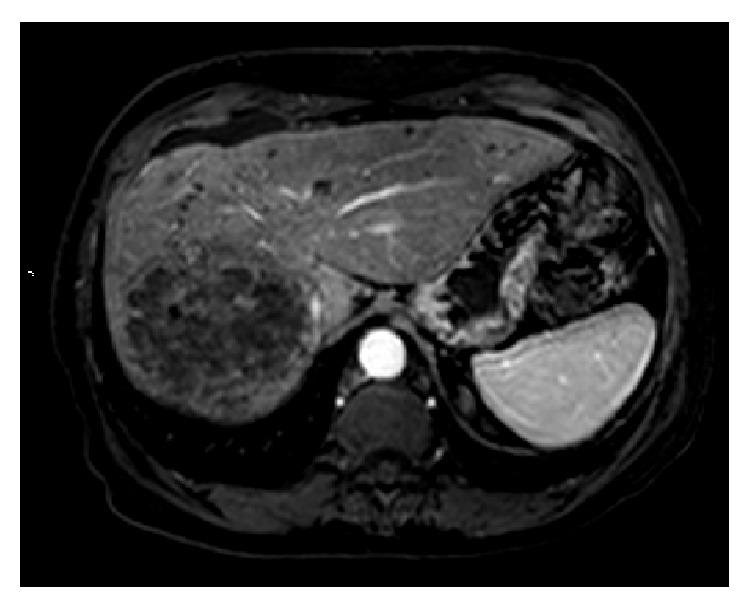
Same lesion on MRI 2 months following radioembolization with yttrium 90 resin microspheres (09/20/2013). There is no enhancement within the central portion of the mass and the tumor has decreased slightly in size, consistent with partial response by mRECIST criteria.
